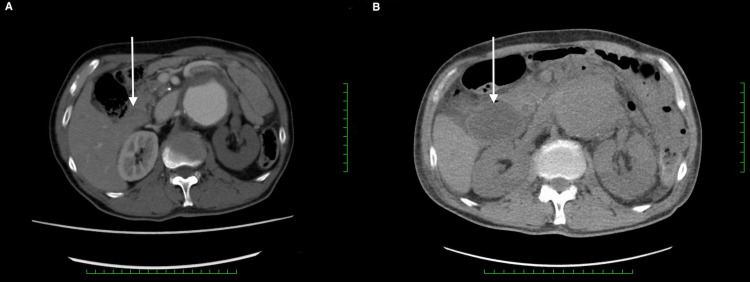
Abdominal CT on admission (A) and day six (B). The duodenum (white arrow) was not dilated on admission (A) but dilated on day six (B). CT: computed tomography
